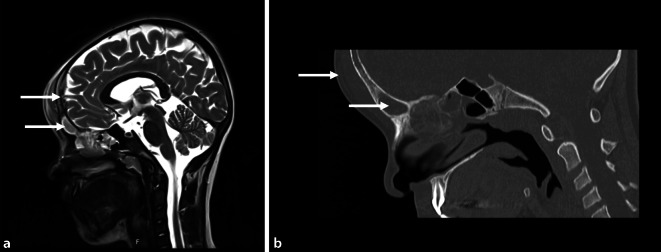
Frontal Pott’s puffy tumor with epidural empyema and frontal/ethmoid sinusitis (*arrows*) (a MRI-, b CT-scan, sagittal)
